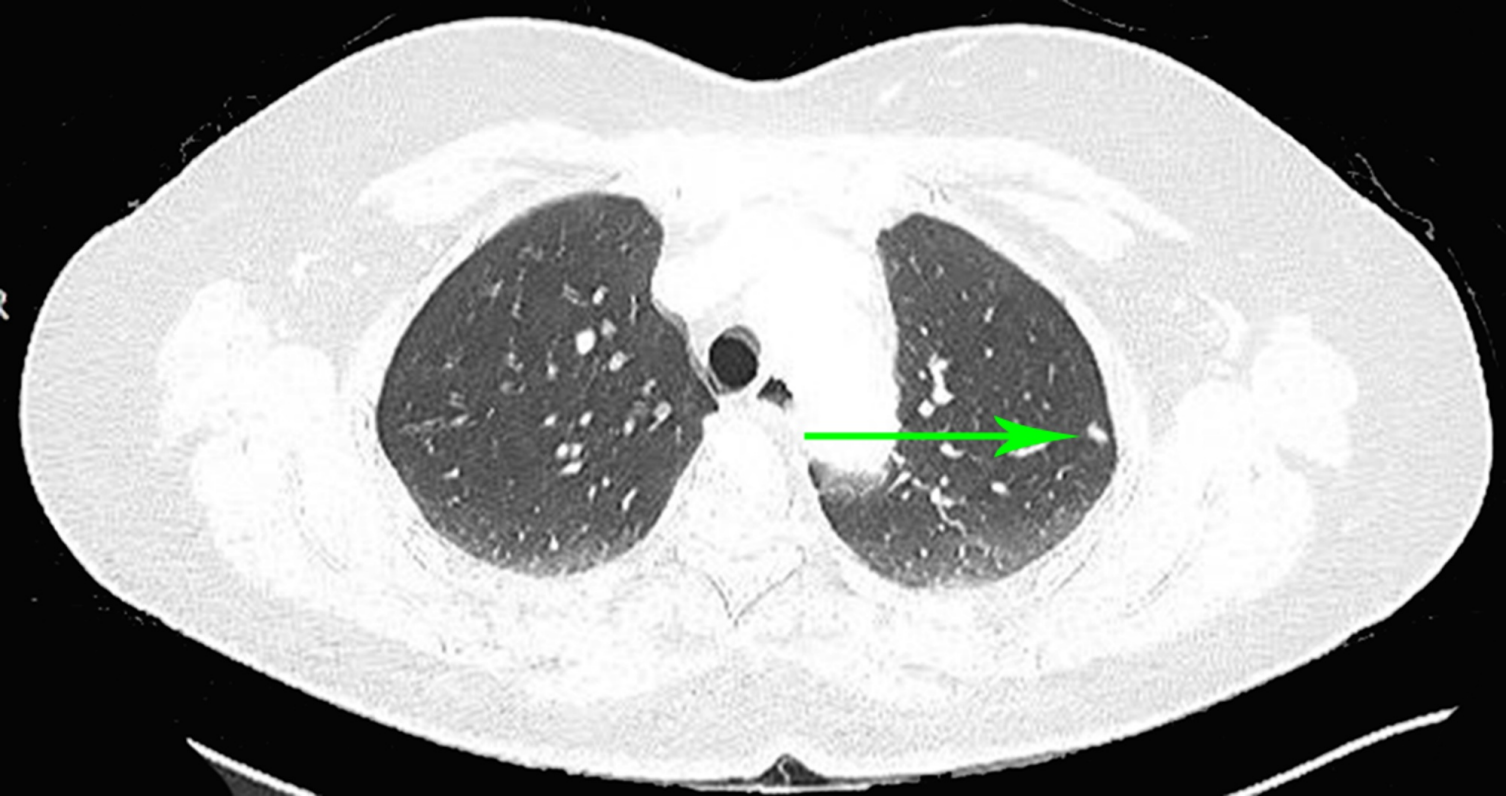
Computed tomography (CT) chest: green arrow pointing at 1.1 cm nodule in the left upper lung lobe.
